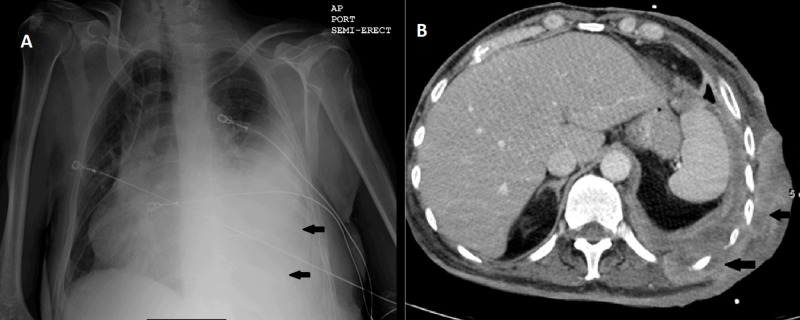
Chest X-ray and axial CT scan (A) Chest X-ray showing left-sided pleural effusion (arrows). (B) Axial CT image showing soft tissue mass invading the chest wall with pleural thickening and effusion (arrows).
